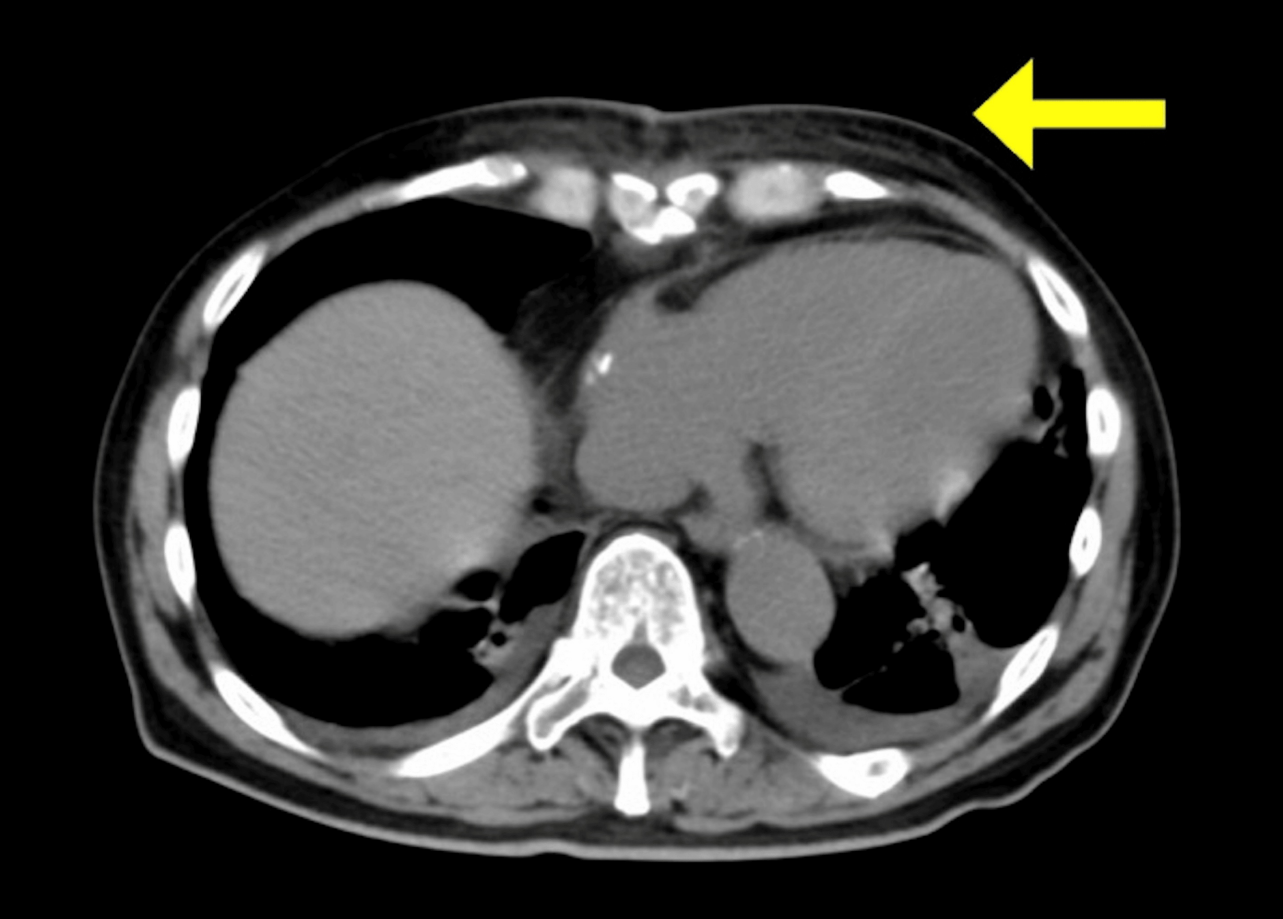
CT on postoperative day 11 A mild increase in fat tissue density is be observed anterior to the sternum
